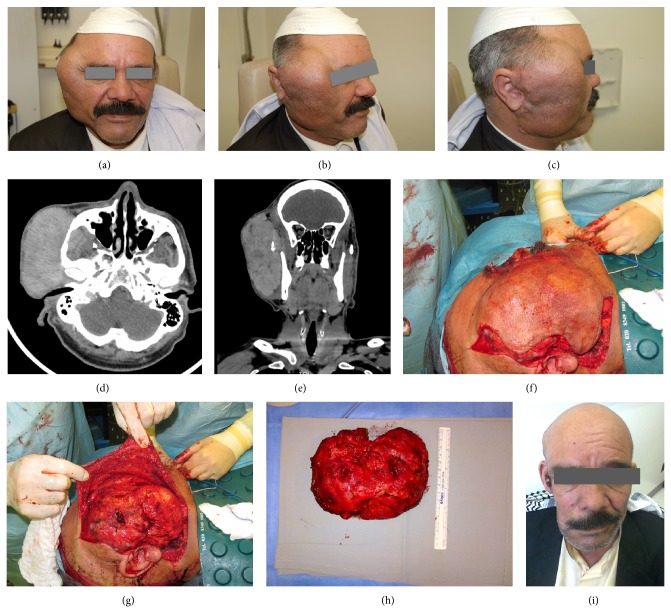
(a) Tumor involving the right cheek area extending from the temporal area to the mandibular area (frontal view). (b) Semilateral view. (c) Lateral view. (d) CT scan: axial cut of the upper neck with IV contrast showing narrowing of the external auditory canal by the tumor. (e) CT scan: coronal cut of the upper neck with IV contrast showing erosion of the right zygomatic arch. (f) Intraoperative image showing an extended modified Blair incision. (g) Intraoperative image showing the parotid mass well circumscribed after elevation of the skin flap. (h) Tumor after “en bloc” excision. (i) Postoperative image of the patient.
